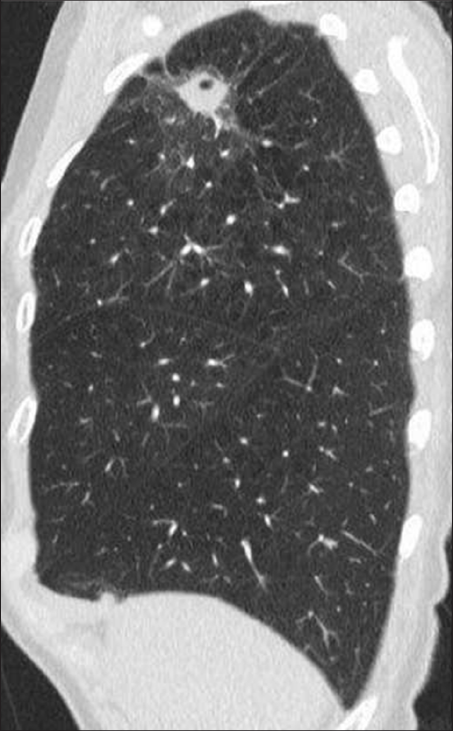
Chronic necrotizing (semi-invasive) aspergillosis*. A 70-year-old female with long-standing asthma was treated with steroids. She was treated for pneumonia but still had rising CRP from 140 to 170mg/L. CT revealed a cavitary nodule in the right upper lobe with ground glass around it, a so-called halo sign (arrows).
